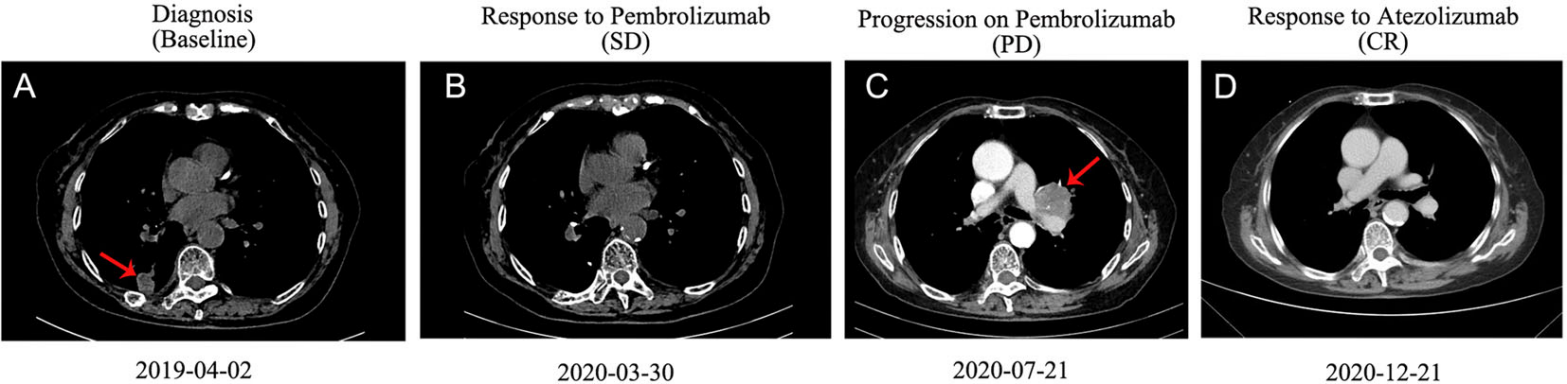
Computed tomography (CT) scans of the thorax of the patient. (A) CT scan before the treatment. (B) Image of the best response of pembrolizumab therapy. (C) Image of progression of pembrolizumab therapy and before atezolizumab therapy. (D) Image of best response of atezolizumab therapy. CR, complete response; SD, stable disease; PD, progressive disease.
